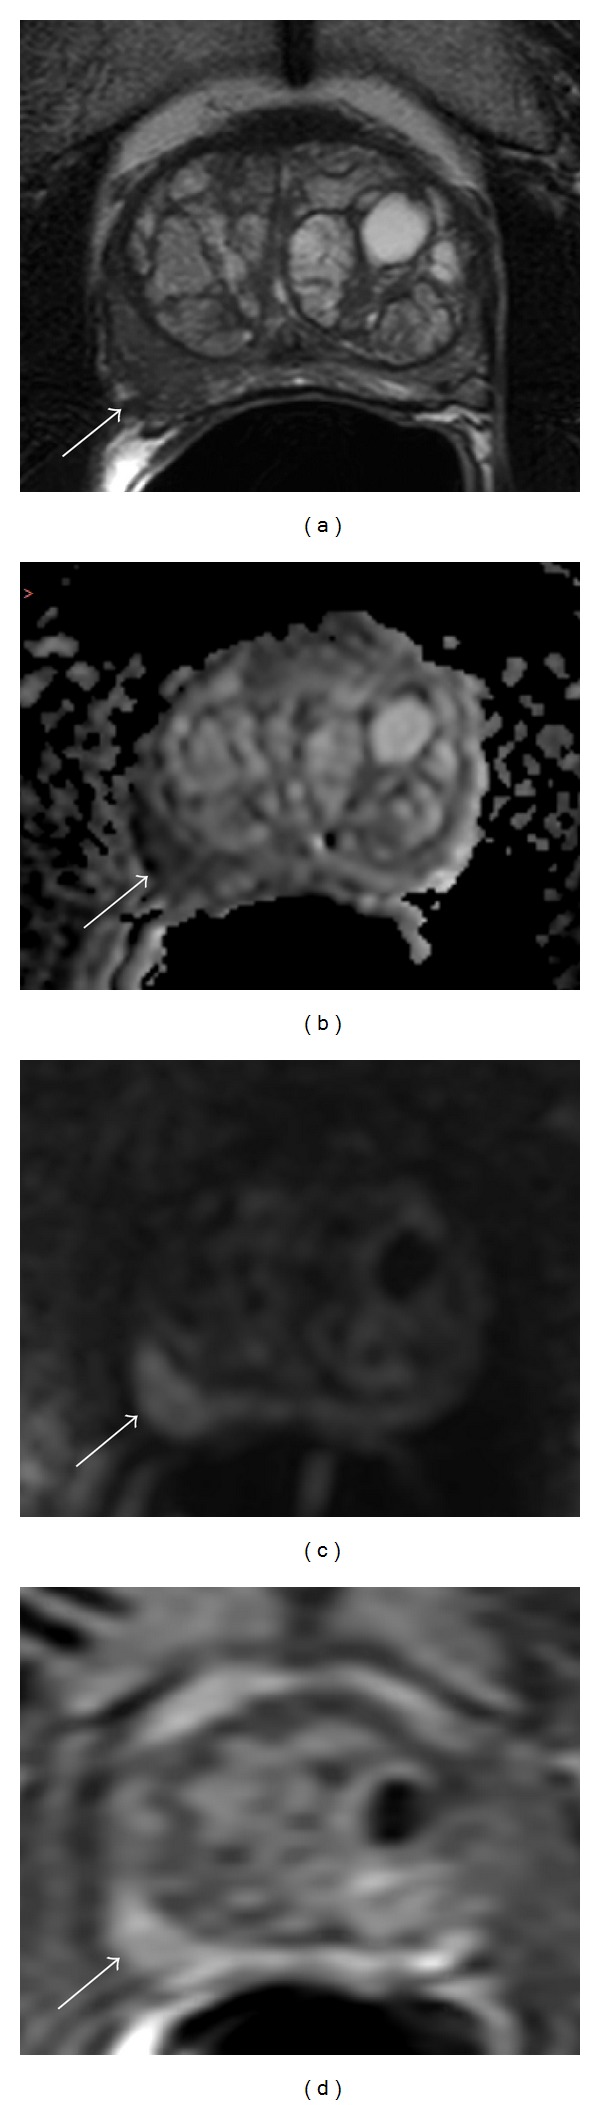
A 58-year-old patient with a PSA of 4.84, previously diagnosed with Gleason 3 + 4 cancer on 12-core template biopsy. Axial T2 weighted image showing hypointense signal in right midperipheral zone with signs of capsular irregularity and suspicion of extracapsular extension (ECE) (arrow) (a). Axial diffusion weighted image showing hypointense area in the right midperipheral zone (arrow) (b). Axial high “*b*” value DWI (*b* = 2000) hyperintense in the right midperipheral zone (arrow) (c). Axial dynamic contrast enhanced image showing strong early enhancement in right midperipheral zone (arrow) (d). Patient underwent an MP-MRI followed by a MRI/transrectal ultrasound (TRUS) fusion guided biopsy upgrading to a Gleason 4 + 3 in all three MP-MRI determined targets of the right midperipheral zone.
